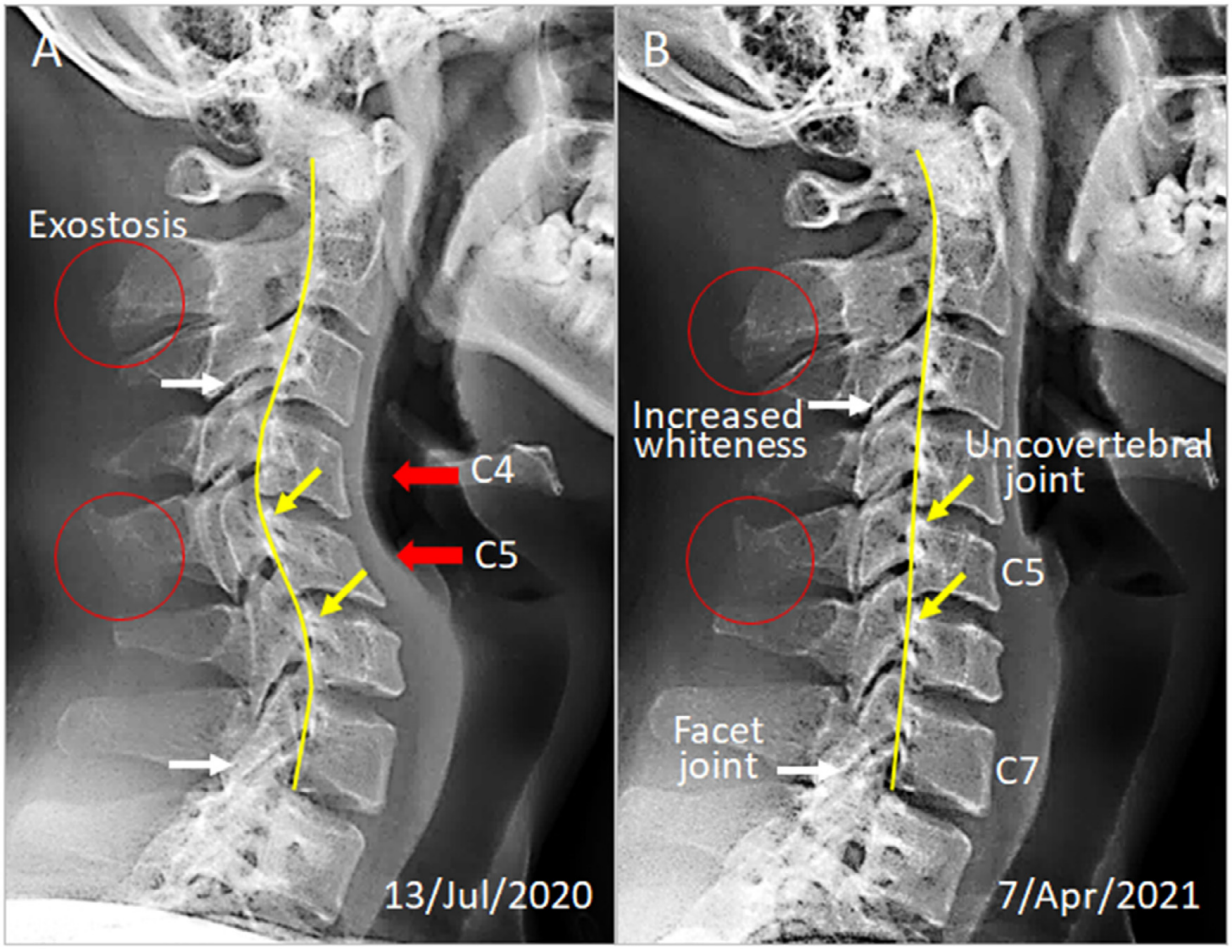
Comparison of cervical alignment on sagittal radiographs. (A) Pretreatment image depicts cervical kyphosis and subluxation of the C4 with relation to C5. Red arrows point to the two segments that are developing reversed curve. Arthritic cervical facet joints and uncovertebral joints are denoted by white and yellow arrows, respectively. Kyphotic angle of the C4/C5 is 23°. The posterior vertebral (yellow) line highlights a distorted curvature. (B) After nine months of treatment, the C5 vertebral rotation was restored, and global cervical curvature returned to 2° lordosis. The radiographic and postural improvement positively correlates with symptom relief.
